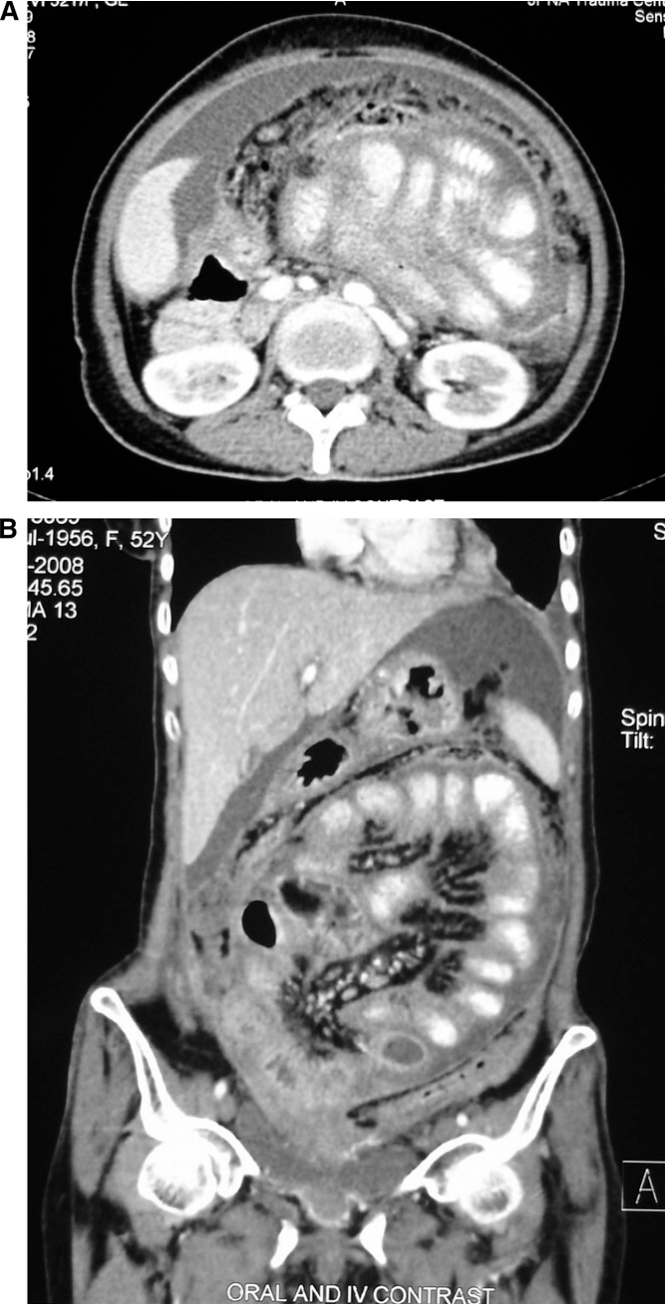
Multi-detector computed tomography (MDCT) of the abdomen axial (A) and coronal reformatted (B) images reveal a 3-mm-thick membrane encasing the small bowel loops and forming a saclike structure.
